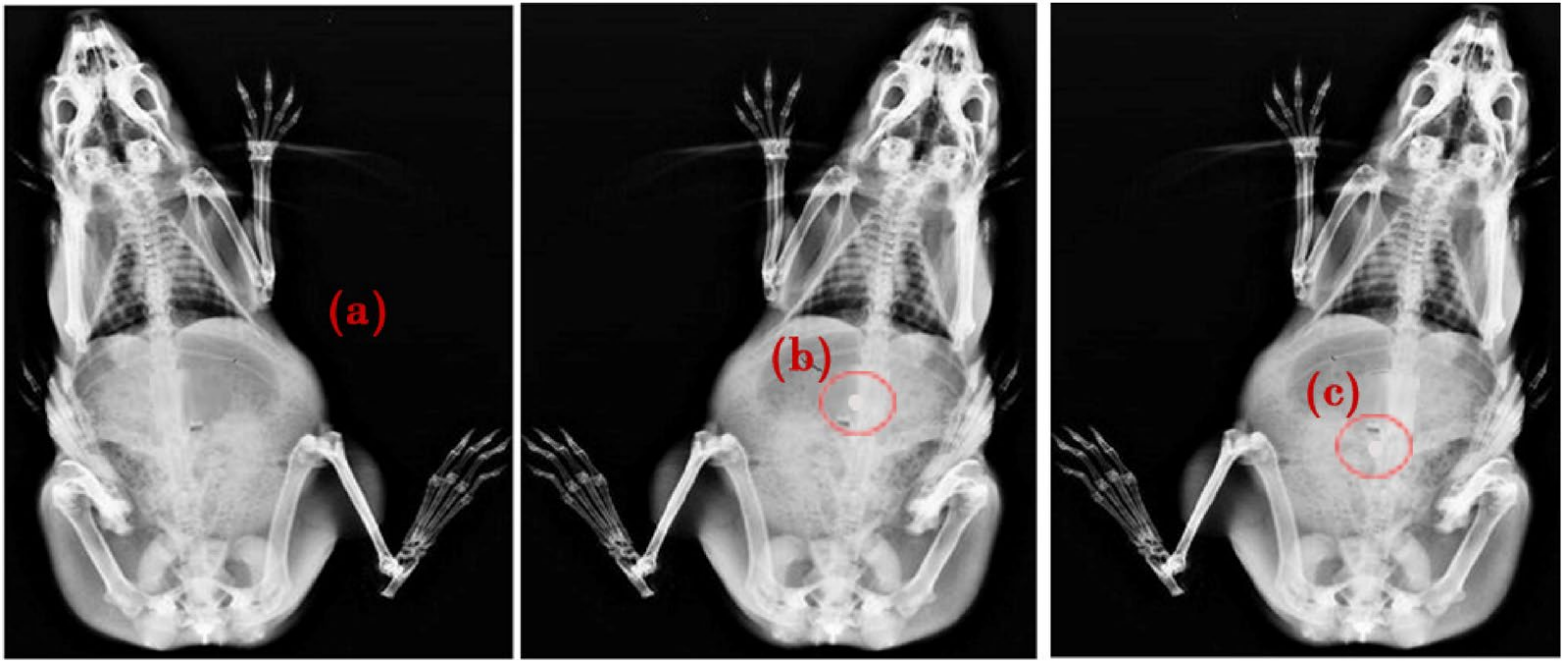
X-ray radiographs showing the location of CCT-KT tablet labeled with a radioopaque marker, Barium sulfate a) Before administration and after b) 2 h, and c) 10 h of formulation administration.
